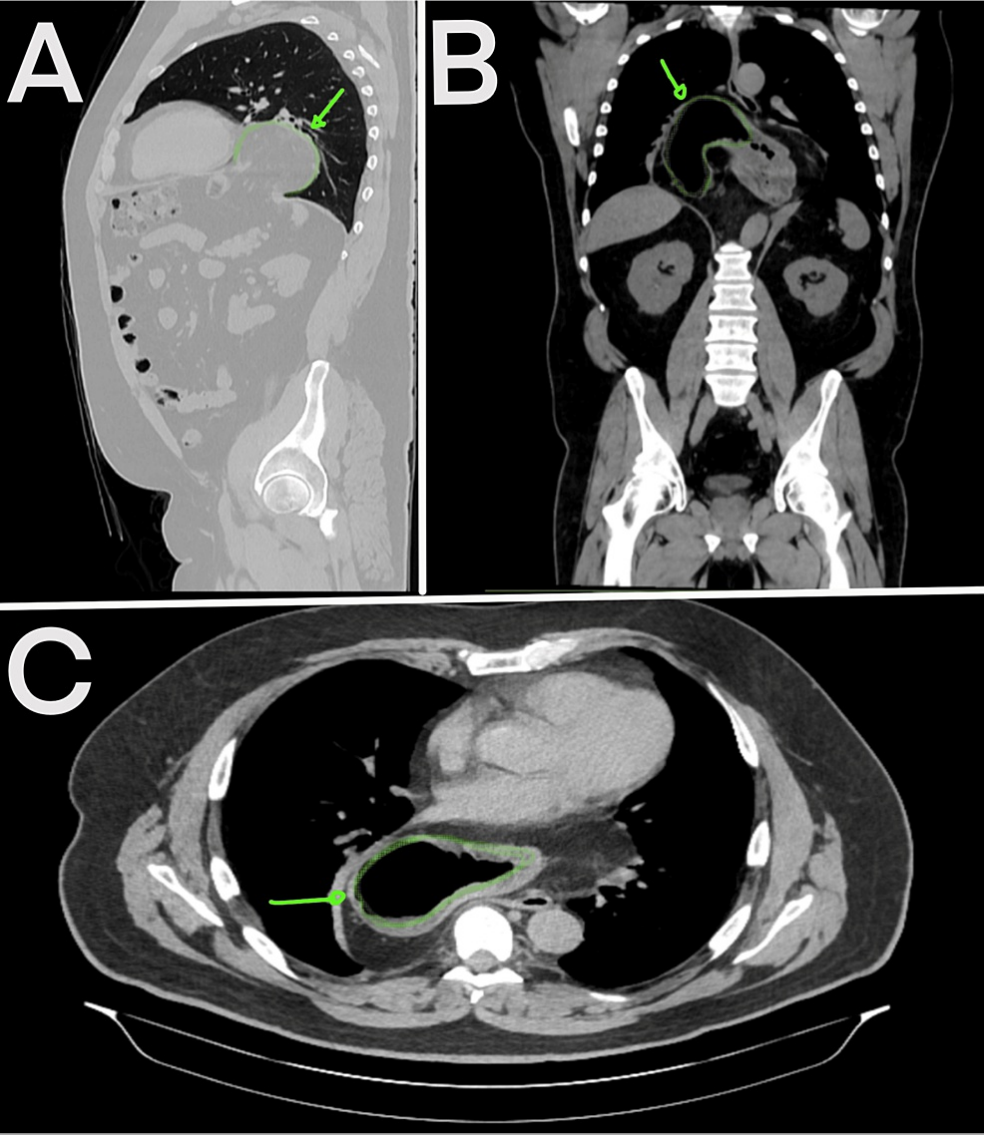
Preoperative computed tomography A: Sagittal view; B: coronal view; C: axial view; the green arrow and green outline indicate the stomach.
